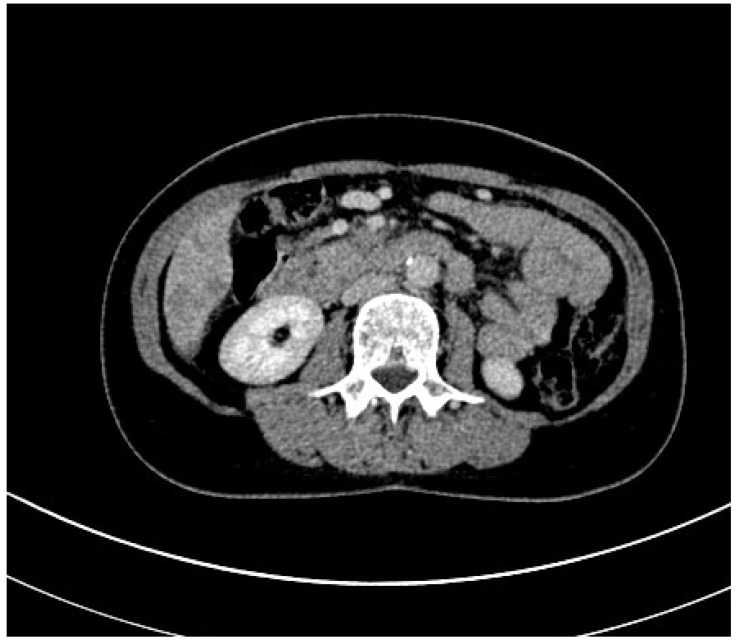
Contrast-enhanced CT scan of a sarcopenic patient with pancreatic carcinoma.
